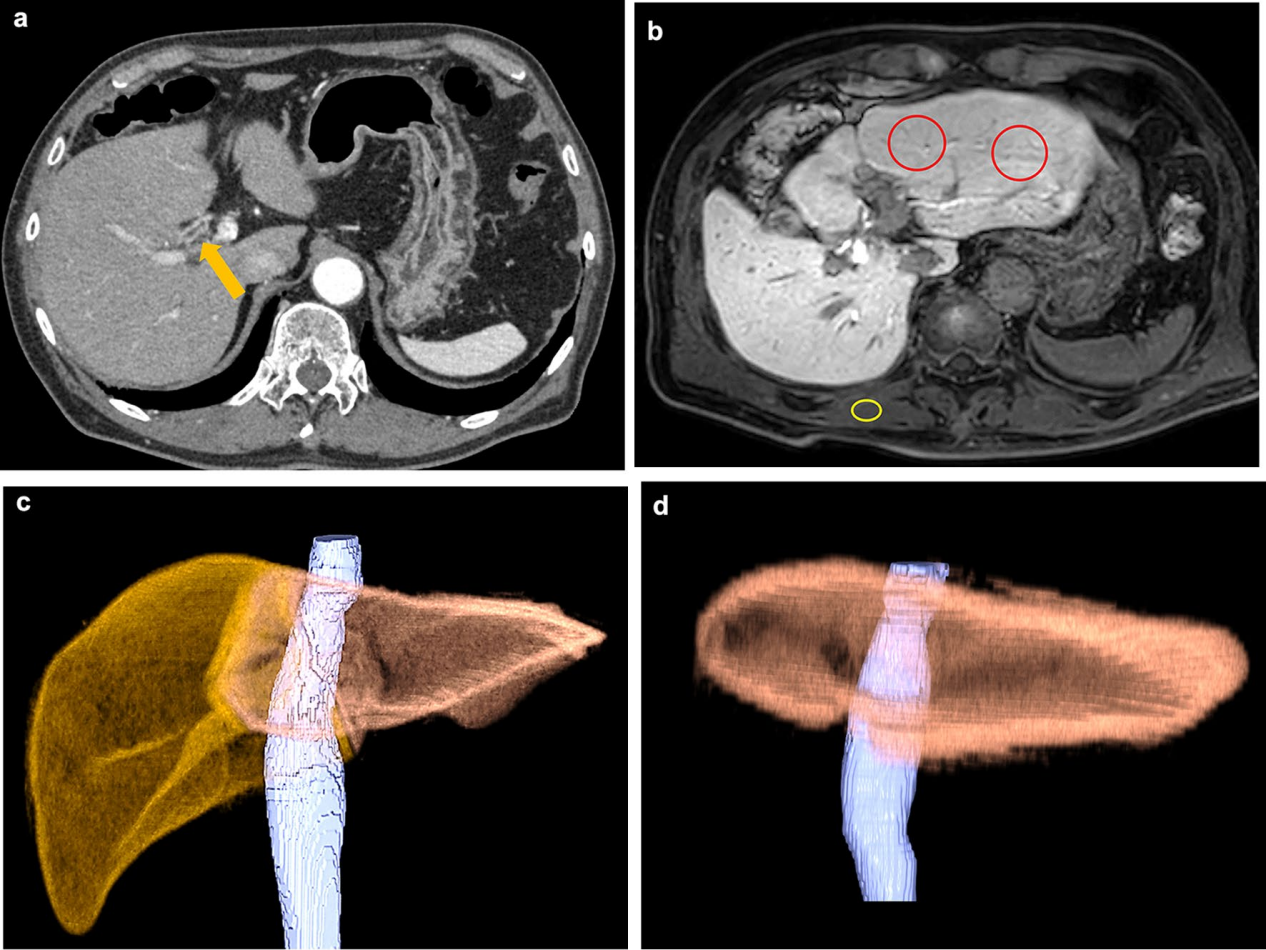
A 79-year-old man with right hilar cholangiocarcinoma. a Arterial-dominant phase of dynamic CT showing wall thickening of the right hepatic duct (arrow). b The hepatobiliary phase of gadoxetic acid-enhanced MRI. ΔLMR of the left lobe was 0.90. c Preoperative CT volumetry. The left and total liver volumes were 368 and 1021 mm^3^, respectively. The resection rate (RR) was 64.0%. d Postoperative CT volumetry was performed 197 days after the right hemihepatectomy. The left liver volume was 915 mm^3^ and the liver regeneration index (LRI) was 148.6%
